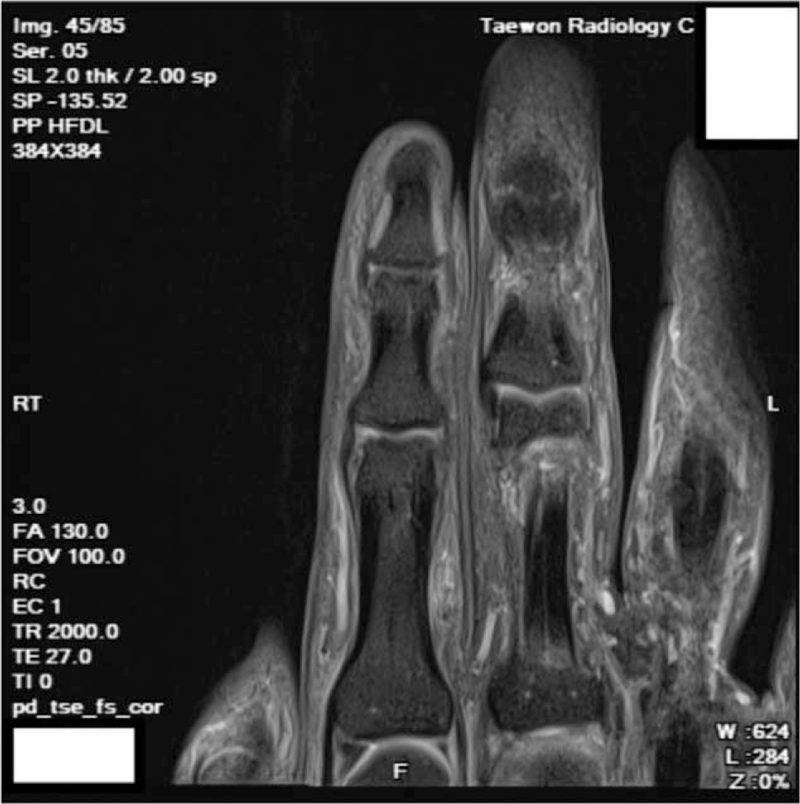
Proton density magnetic resonance image of the right second distal interphalangeal joint.
